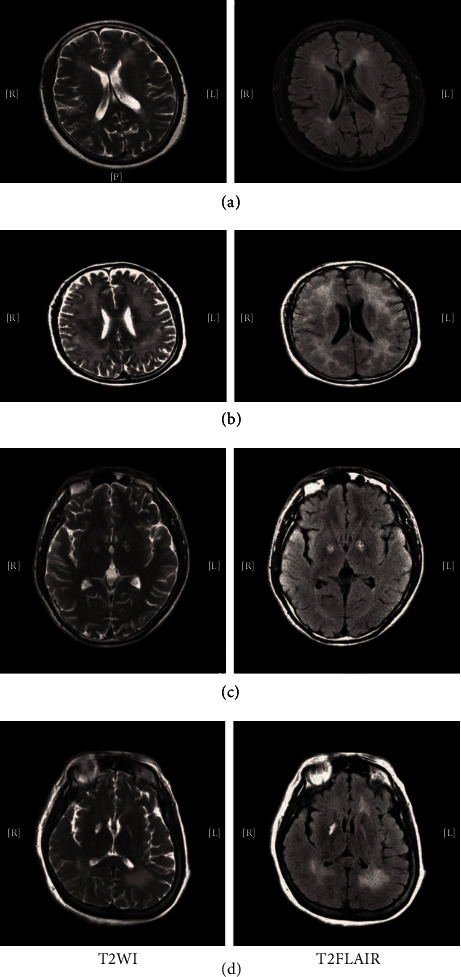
magnetic resonance imaging (MRI) scan showed high signal intensity in the bilateral cerebral white matter (a, b) and the globus pallidus(c, d).
